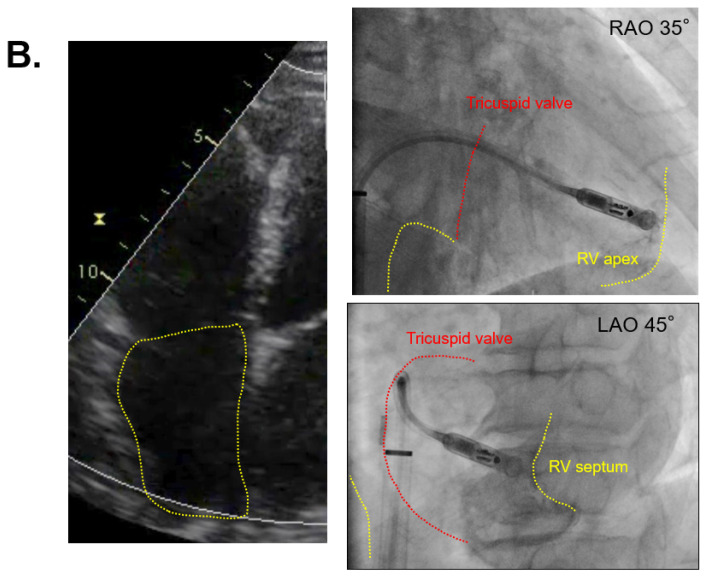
Echocardiography in the shortest and longest procedures. (A) The case of smaller right atrium with 30 min of procedure time; (B) the case of larger right atrium with 185 min of procedure time; echocardiographic views indicate four chambers, viewing from the apex, and yellow dashed lines indicate the right atrium; IVC = inferior vena cava, RV = right ventricle.
